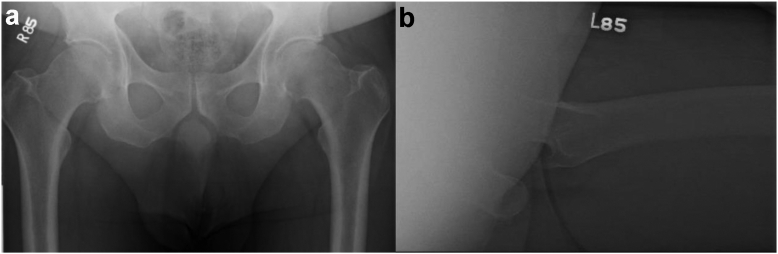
Preoperative left primary THA radiographs. (a) Anteroposterior (AP) pelvis and (b) left lateral radiographs demonstrate no acute fracture or bony malalignment. The left hip shows severe degenerative changes with joint space narrowing in addition to a small focus of superior femoral head avascular necrosis with collapse.
